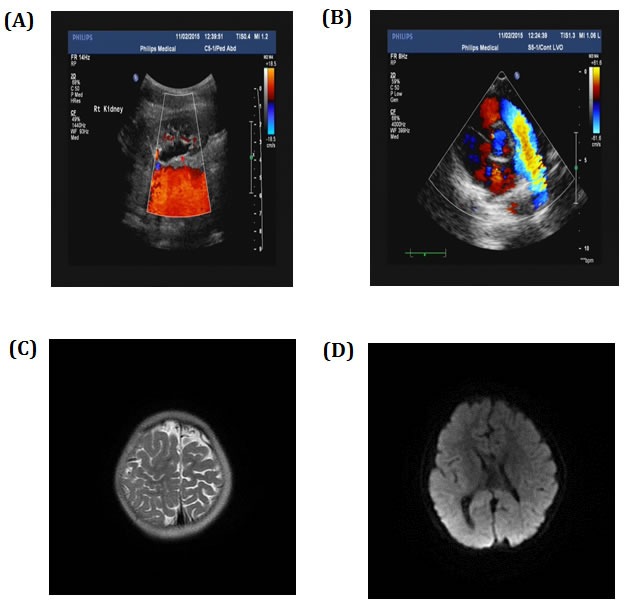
Ultrasonography showed A. double kidney effusion and B. normal heart. CT scan showed C. right side schizencephaly and D. callosal agenesis.
